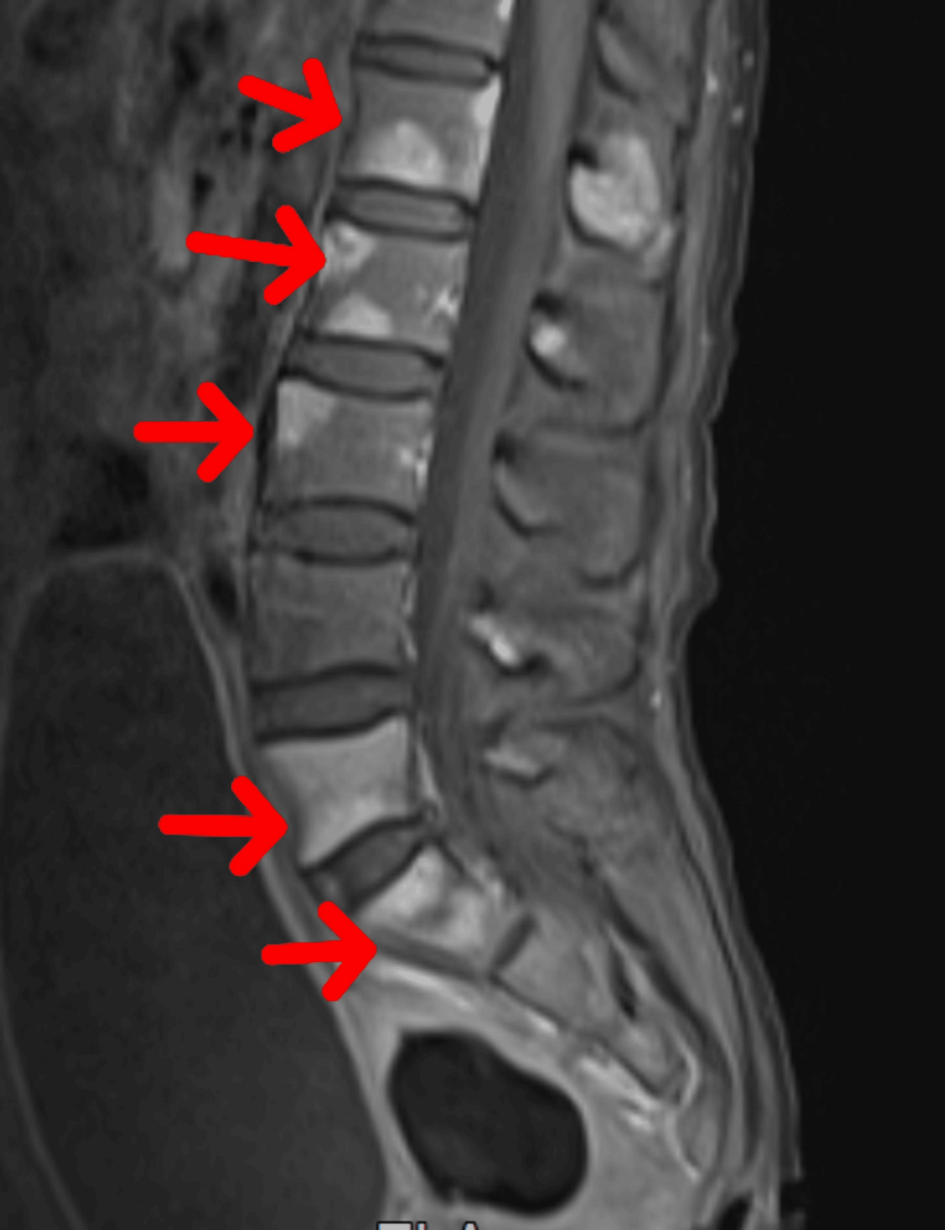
The spinal MRI: multiple vertebral lesions with hyperintensity on fat-suppressed T2-weighted imaging (arrows)
